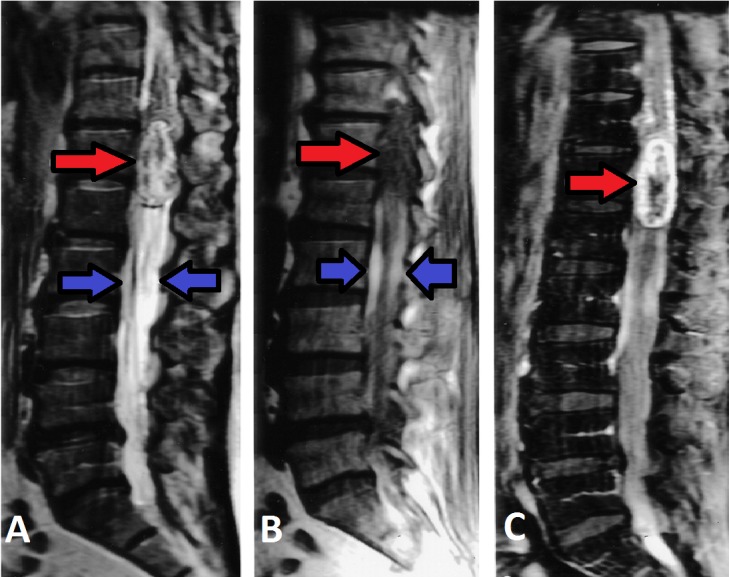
MRI of thoracolumbar spinal tumor Sagittal T2- [A] and T1-weighted [B] imaging shows a heterogeneous signal intensity of large mass at the conus medullaris (red arrows). The two hyperintense subdural hematomas located caudal to the mass (blue arrows). There is heterogeneous enhancement of the mass post-intravenus contrast [C]
